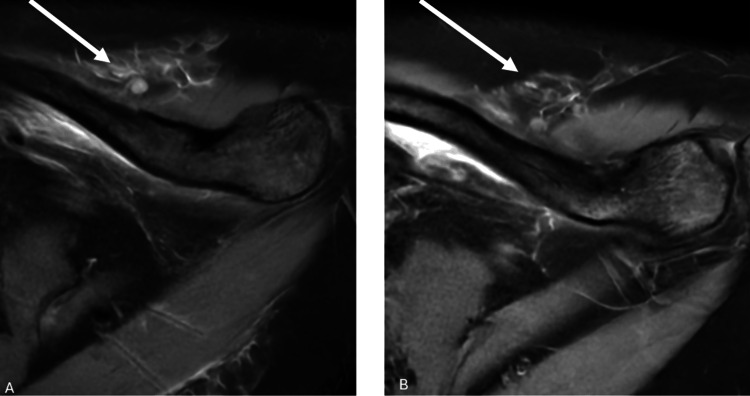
(A-B) Axial magnetic resonance images of the patient. The white arrow shows the lesion in the relevant magnetic resonance images.
